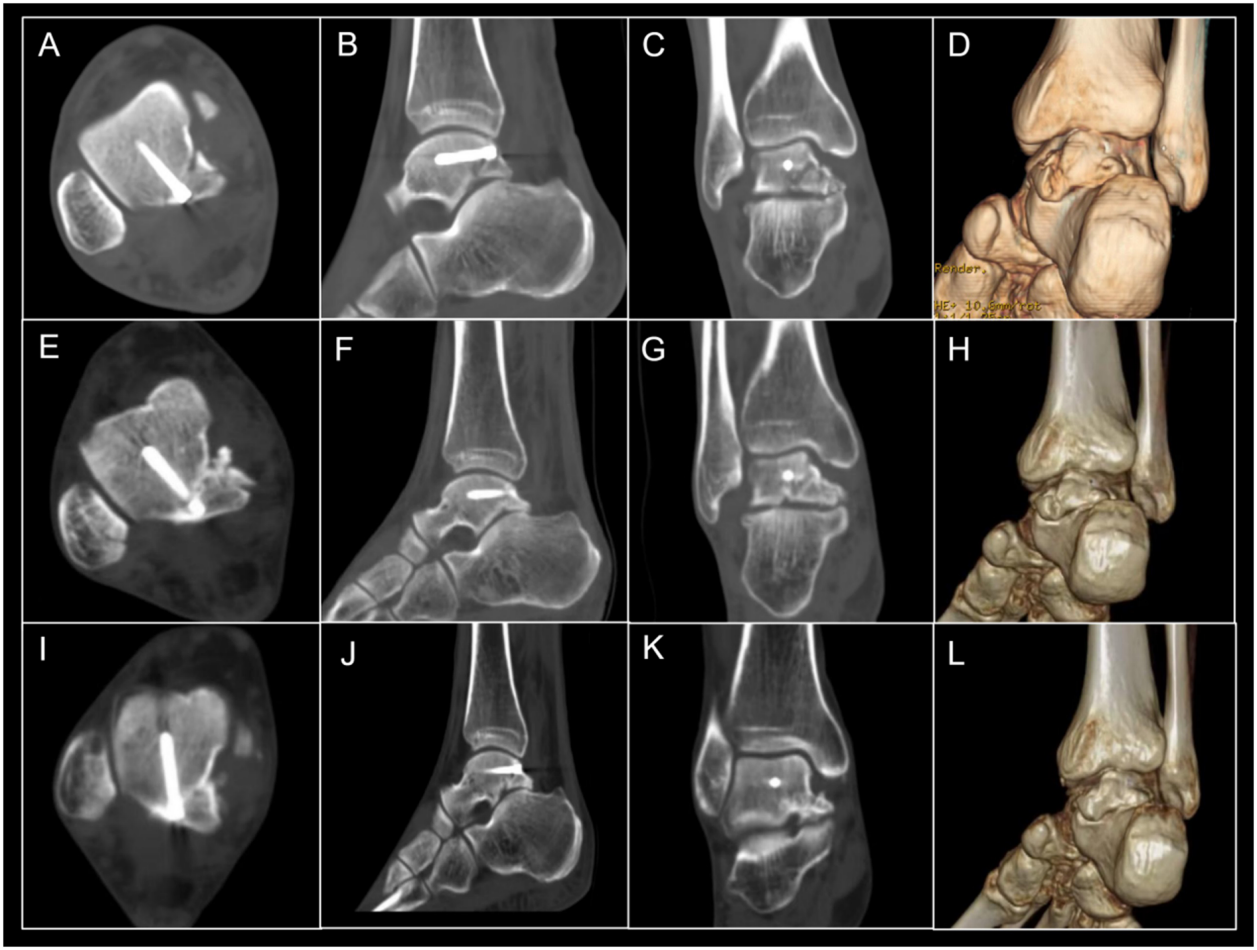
CT scan (A–D) 2 days postoperatively showing good fixation; CT scan (E–H) 2 months postoperatively showing signs of healing in the fixed fracture fragment; CT scan (I–L) 2 years postoperatively showing favourite fracture healing, with improved healing of the posterior lateral tubercle.
